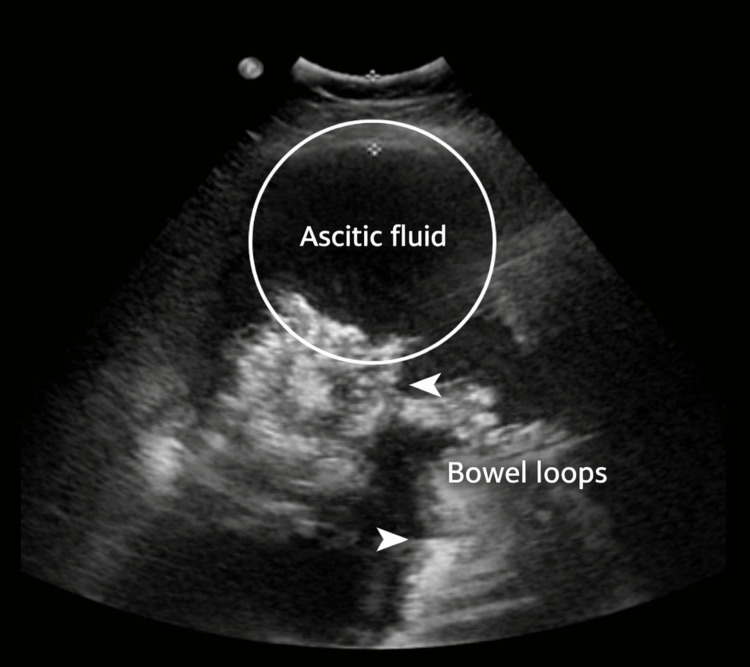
Ultrasound-guided paracentesis Transabdominal ultrasound of the left lower quadrant demonstrating a large anechoic pocket of intraperitoneal fluid consistent with ascites (circled). The surrounding echogenic structures correspond to displaced bowel loops within the peritoneal cavity. The fluid-filled space provided an appropriate window for ultrasound-guided paracentesis.
